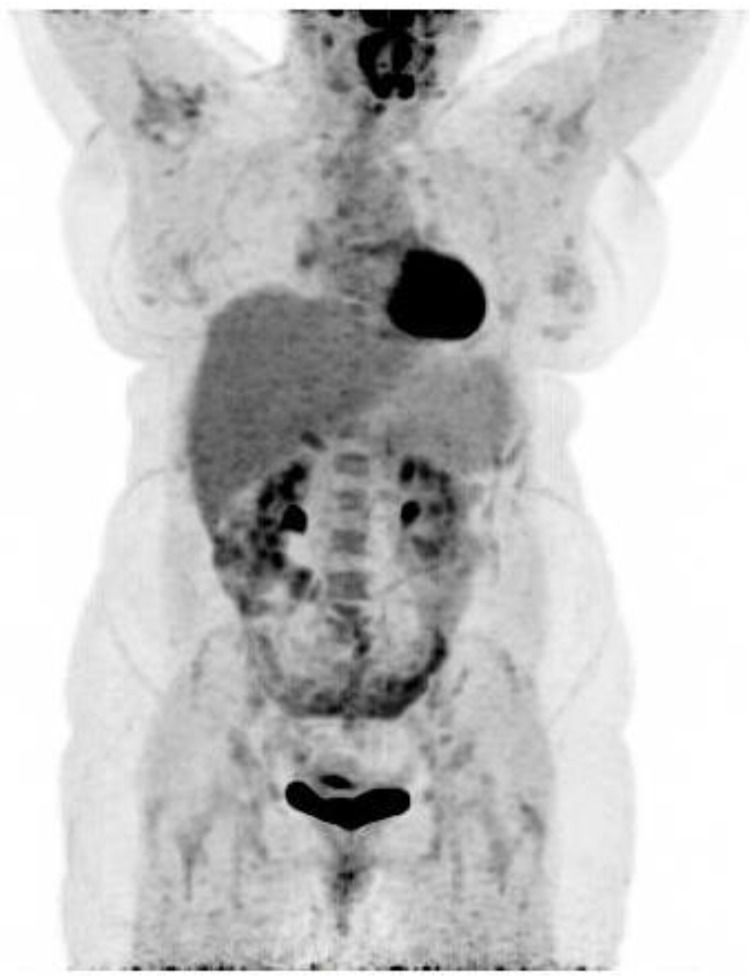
In the meantime, additional diagnostic work-up performed two days prior to the ^18^F-FDG PET/CT, came back with positive Chikungunya IgG and IgM serology. Given the patient’s recent travel to India, the clinical course and positive Chikungunya serology, the patient’s abnormal ^18^F-FDG PET/CT scan was most likely secondary to acute Chikungunya infection and no lymph node biopsy was performed or further evaluations considered. After 12 weeks of gradually decreasing dosage of prednisolone the patient returned to her job as a cleaning lady, but was still reliant on pain-medication. A follow-up ^18^F-FDG PET/CT scan 10 days after ending steroid treatment and 14 weeks after the first ^18^F-FDG PET/CT, showed complete regression of the reactive lymph nodes as well as the ^18^F-FDG uptake in the left breast and only discrete ^18^F-FDG uptake of the right shoulder joint, consistent with the patient’s clinical improvement. Any lingering suspicion of underlying lymphoma was therefore rebuked. A needle biopsy from the left breast showed chronic inflammation but no malignancy. The Chikungunya virus is transmitted by the mosquito vector *Aedes aegypti* and *albopictus* in many parts of the world, including India. Chikungunya viral infection is characterised by flu-like symptoms with high grade fever, headache, myalgia, polyarthralgia (usually symmetrical arthralgia, involving multiple joints) and often accompanied by a maculopapular rash [1]. Severe prolonged arthralgia months to years after infection is a recognised post-infectious manifestation of Chikungunya infection. Symptoms of acute Chikungunya are most likely caused by direct cellular damage and local inflammation with muscles, joints, lymph nodes, skin, liver and spleen as primary sites of replication. Chronic disease seems to be mediated by persistent virus and inflammation [2]. To our knowledge, ^18^F-FDG uptake in a case with confirmed Chikungunya infection has not previously been reported. The ^18^F-FDG PET/CT scan confirmed inflammation of several joints and lymph nodes four weeks after the patient’s initial symptoms. Changes that regressed almost completely. In one report, an Indian patient with suspected Chikungunya infection had ^18^F-FDG uptake in lymph nodes on both sides of the diaphragm including splenic activity and active subcutaneous nodules. However, lymph node biopsy revealed angioimmunoblastic/peripheral T-cell lymphoma [3]. Recently, increased ^18^F-FDG uptake in the spleen and multiple lymph nodes was detected in a patient diagnosed with dengue fever and ^18^F-FDG has been suggested to be a novel Dengue infection-associated inflammation biomarker for assessing treatment response during therapeutic intervention trials [4,5]. ^18^F-FDG PET/CT may be of value in assessing patients suspected for long-term morbidity of Chikungunya infection due to persistent arthralgia.
